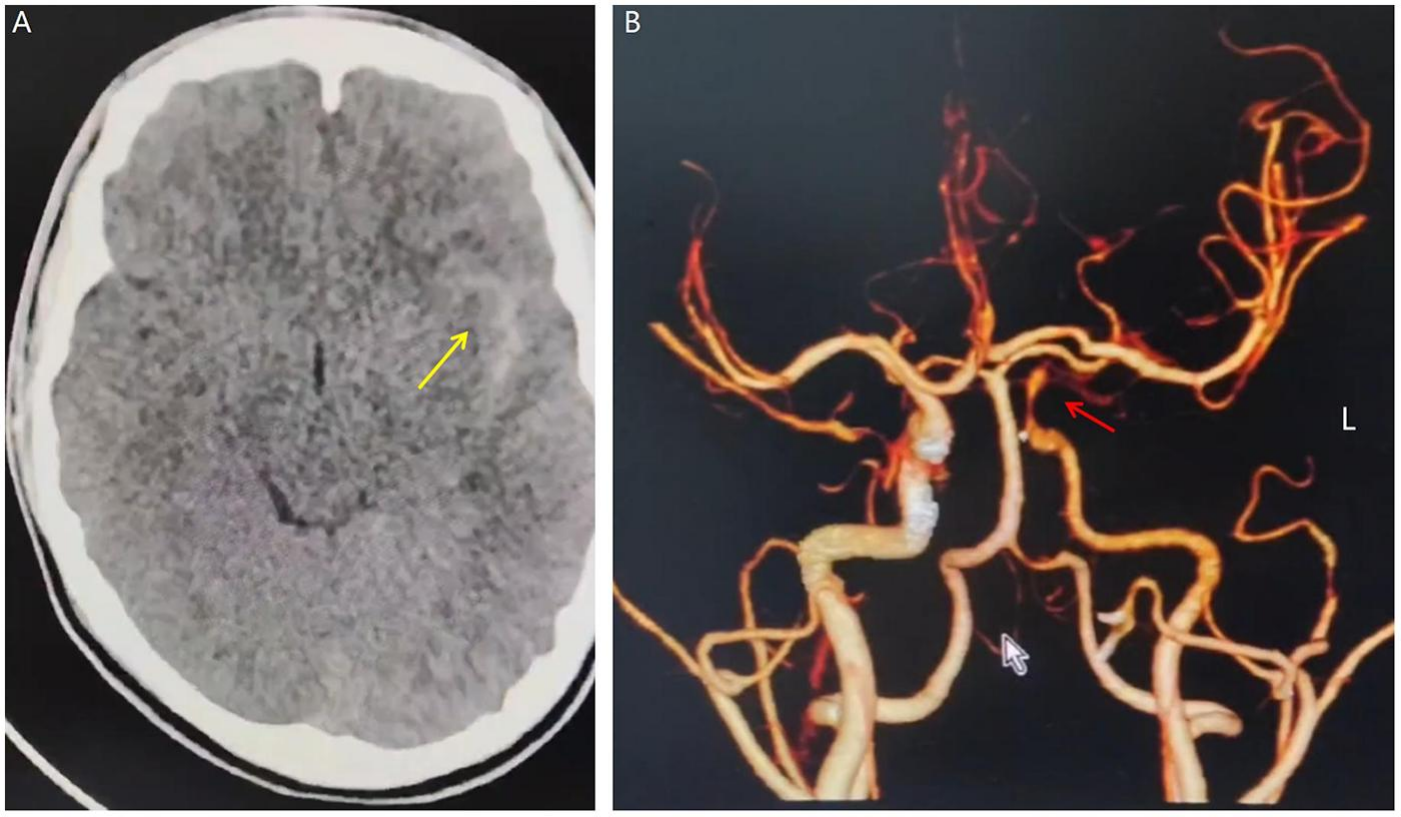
(A) Non-contrast cranial CT demonstrates subarachnoid hemorrhage in the left Sylvian fissure (yellow arrow). (B) Three-dimensional CTA reconstruction reveals segmental stenosis at the communicating segment of the left internal carotid artery (ICA), suggestive of a dissecting aneurysm (red arrow).
